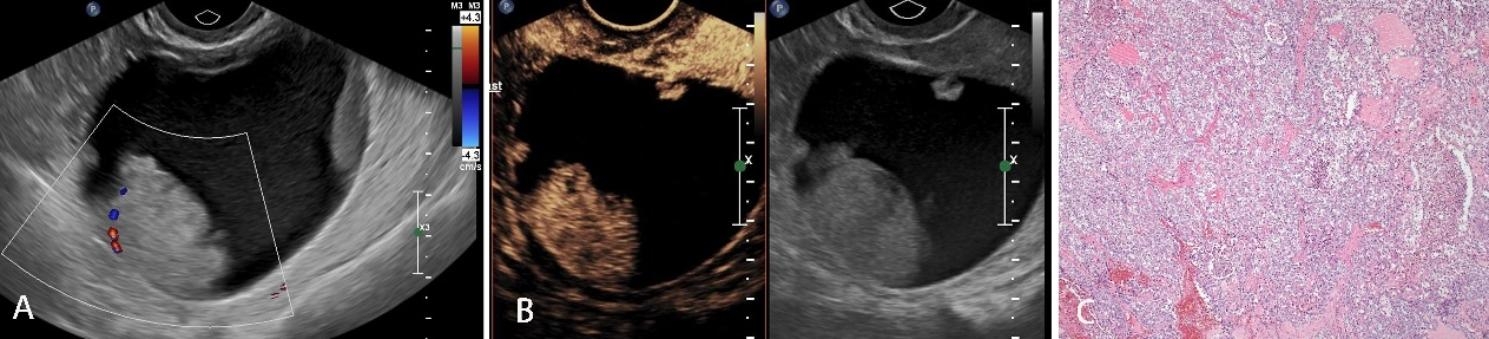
Transvaginal ultrasonogram and pathological image of left adnexal mass in a 50-year-old patient. A: Conventional ultrasound showed a regular unilocular cyst with a maximum diameter 8.8 cm, with three solid nodules. CDFI showed a little blood flow signals at the rim of the largest nodule. The mass was classified as 4 by O-RADS. B: CEUS showed annular rim enhancement at 33s, and all the internal nodules displayed nonhomogeneous hyperenhancement; then, it was adjusted to 5 by O-RADS combined with CEUS. C: Pathological results confirmed it as left ovarian clear cell carcinoma (HE × 40)
